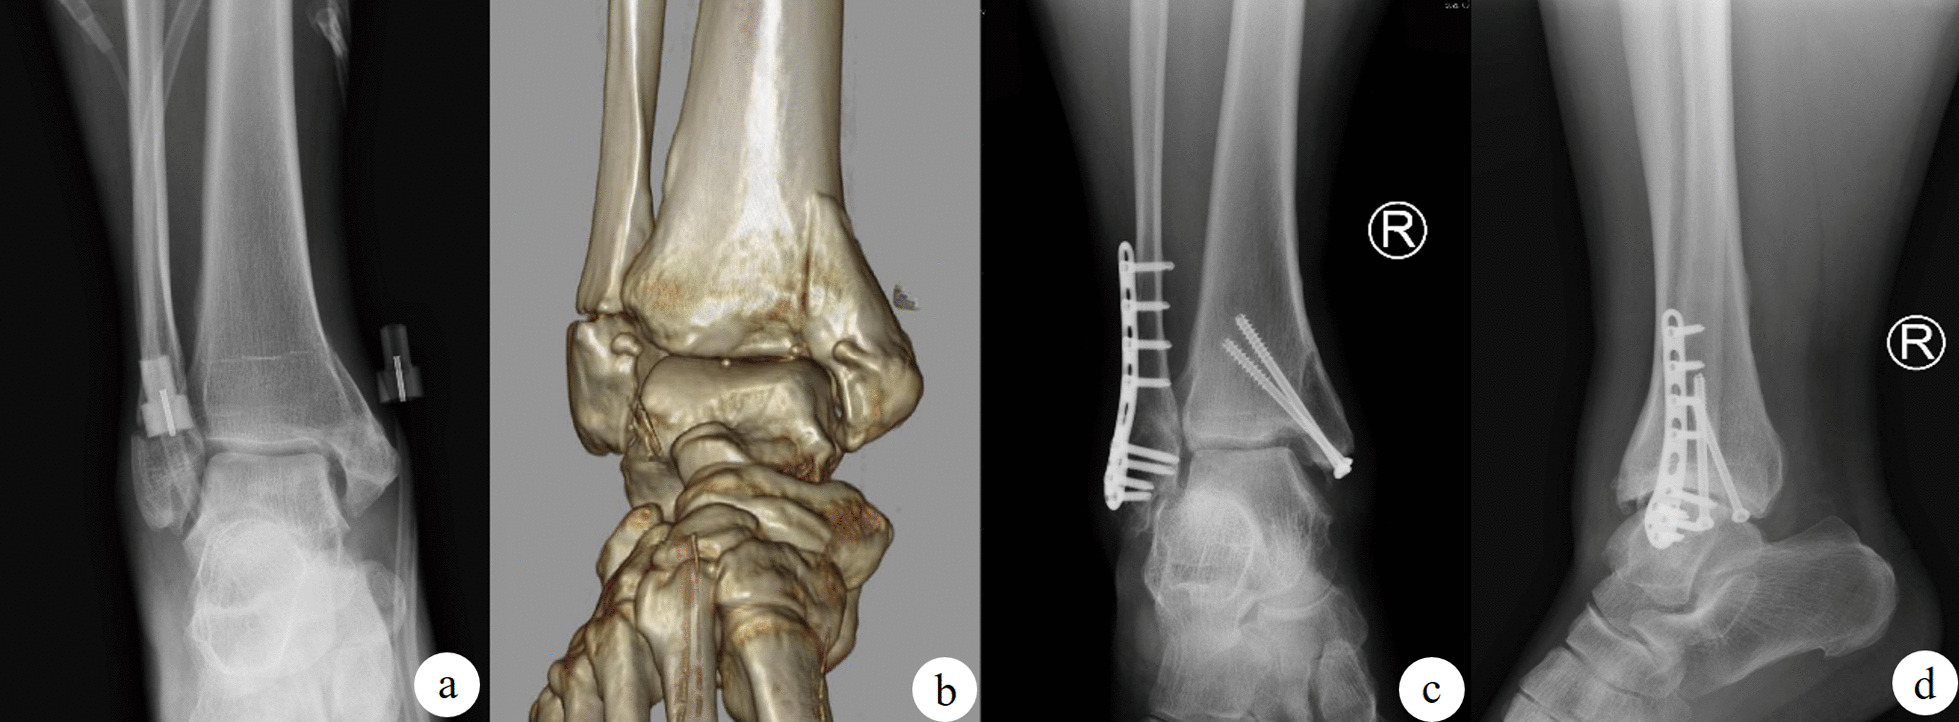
The use of screws to fix medial malleolar fractures is a classic fixation method. a The orthographic X-ray images of the fracture; b the CT three-dimensional reconstruction image after the fracture; c, d the orthographic X-ray images after screw internal fixation
